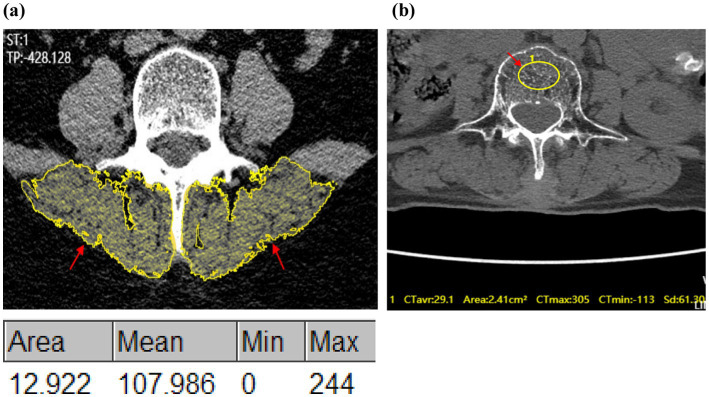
The measurements of muscle area (A) and bone value (B). The bone value and the area of the paraspinal muscles on both sides were measured on axial CT images at the medium level of the L4 vertebrae.
